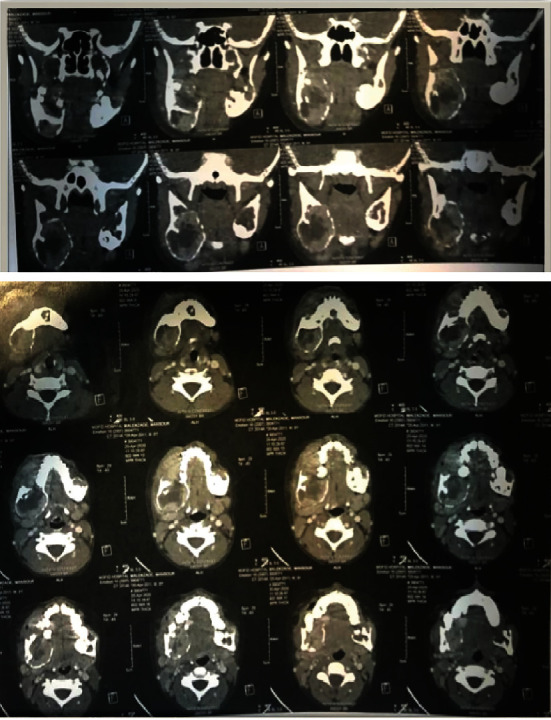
The coronal (above) and axial sections (below) of post-contrast CT.
